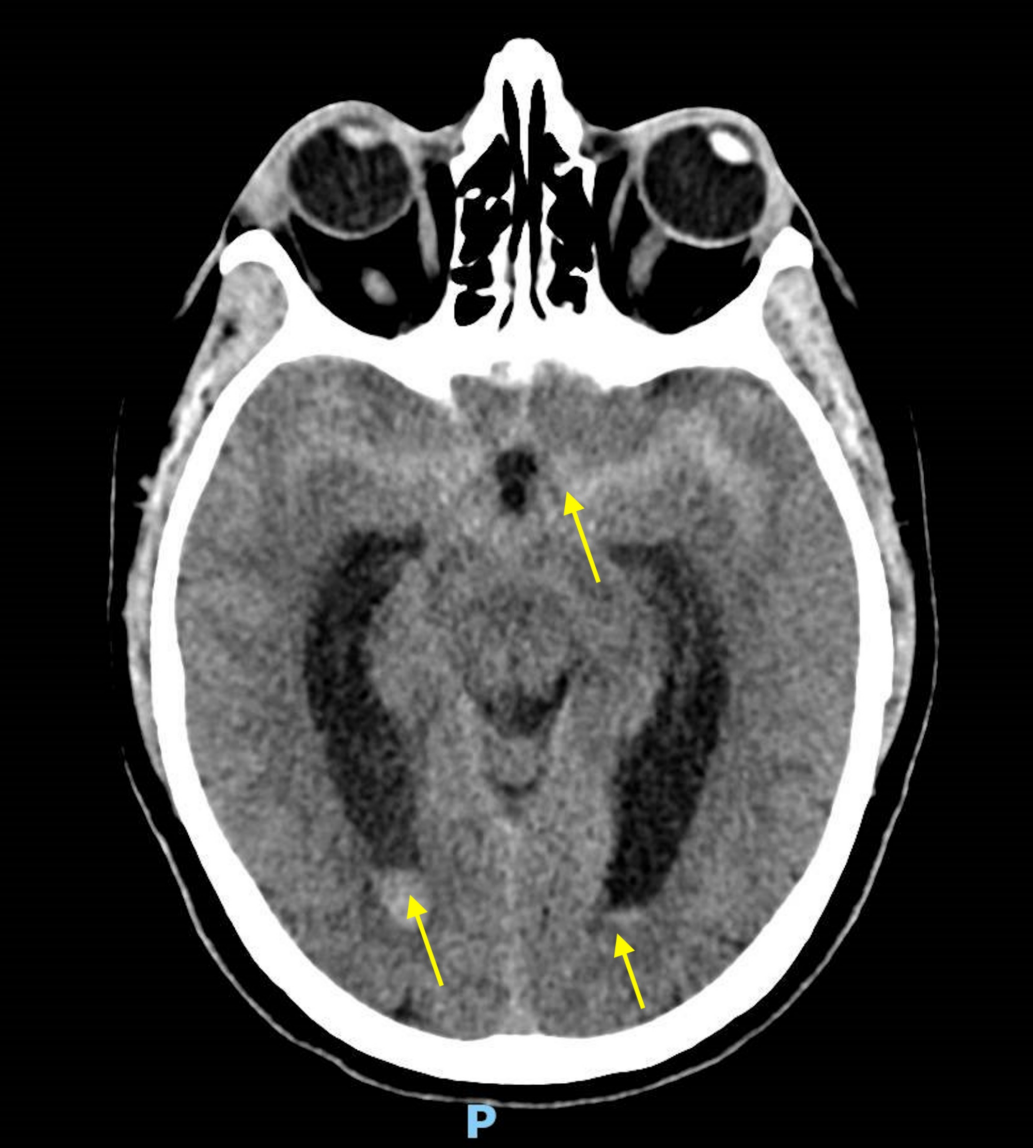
CT imaging demonstrated a subarachnoid hemorrhage of Fisher grade 4 (yellow arrows demonstrate hypodense regions of subarachnoid and bilateral intra-ventricular blood).
